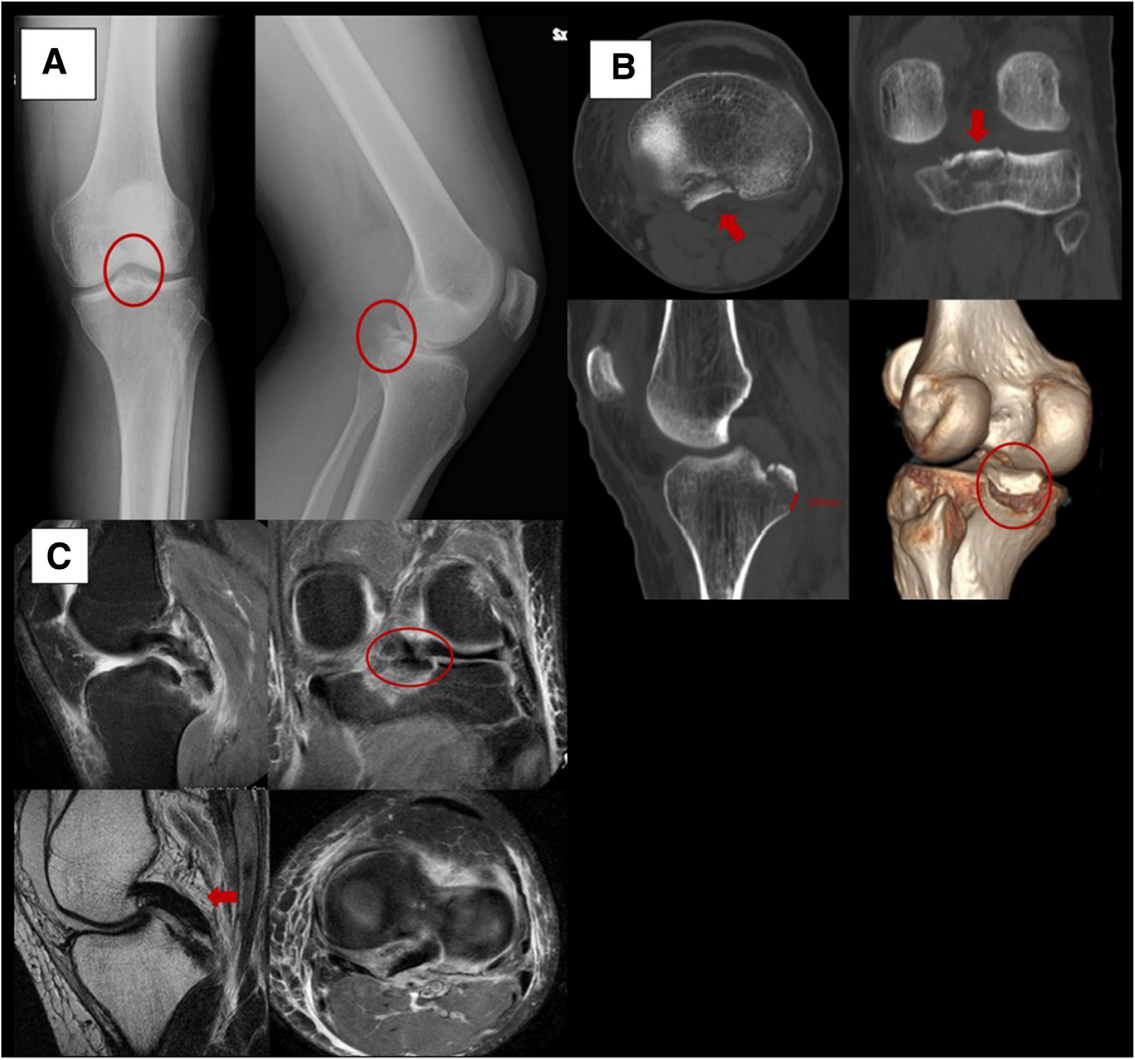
Preoperative imaging of left knee. (A) Anteroposterior and lateral radiographs showing isolated posterior cruciate ligament (PCL) avulsion fracture (ovals). (B) Axial, coronal, sagittal, and 3-dimensional computed tomography scans of same PCL avulsion fracture. Computed tomography reveals a multifragmentary fracture (arrows and red circle) with a major fragment and displacement of 10 mm, which requires surgery. (C) Sagittal, coronal, and axial magnetic resonance imaging (MRI) of same isolated PCL avulsion fracture. MRI reveals the continuity of the PCL substance signal (arrow) and edema at the fracture level (oval). MRI enables the identification of any associated meniscal or ligamentous lesions, as well as intra-articular free bodies.
